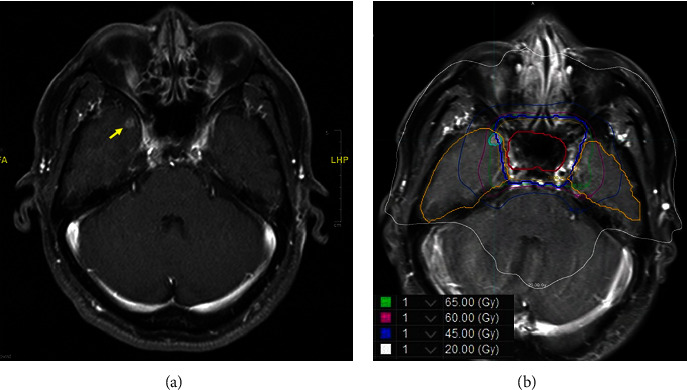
Magnetic resonance imaging (MRI) showed (a) a small enhancing focal lesion in the right temporal pole with a size of 9 mm × 7 mm, and brain radiation necrosis was suspected (arrow indicates the lesion with rim enhancement). (b) Superimposition of cumulative radiation dose distribution on transverse postcontrast MRI showing the relation between the radiation dose and lesion with rim enhancement (indicated with aqua).
